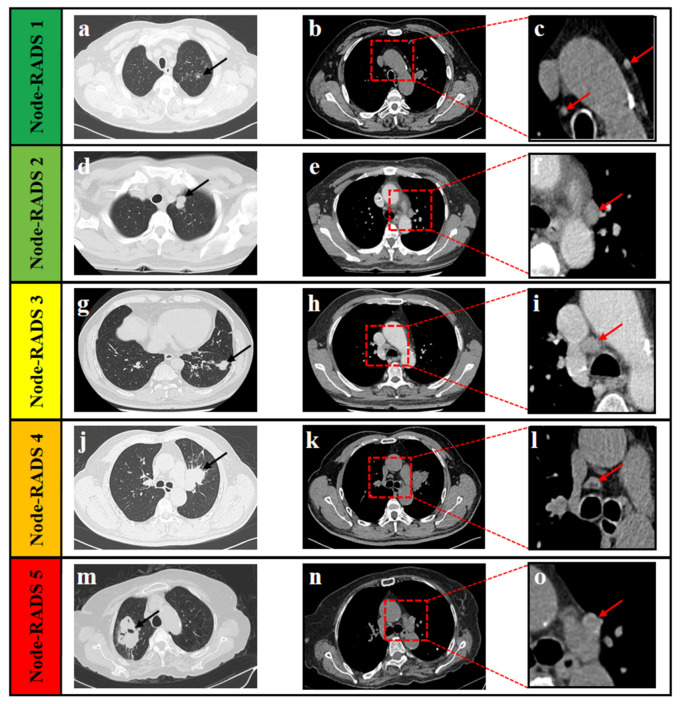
Representative CT images of Node-RADS categories 1–5. Black arrows indicate primary tumors; red boxes indicate selected regional lymph nodes; red arrows indicate magnified lymph-node views. Images (a–c), (d–f), (g–i), (j–l), and (m–o) correspond to Node-RADS categories 1, 2, 3, 4, and 5, respectively.
